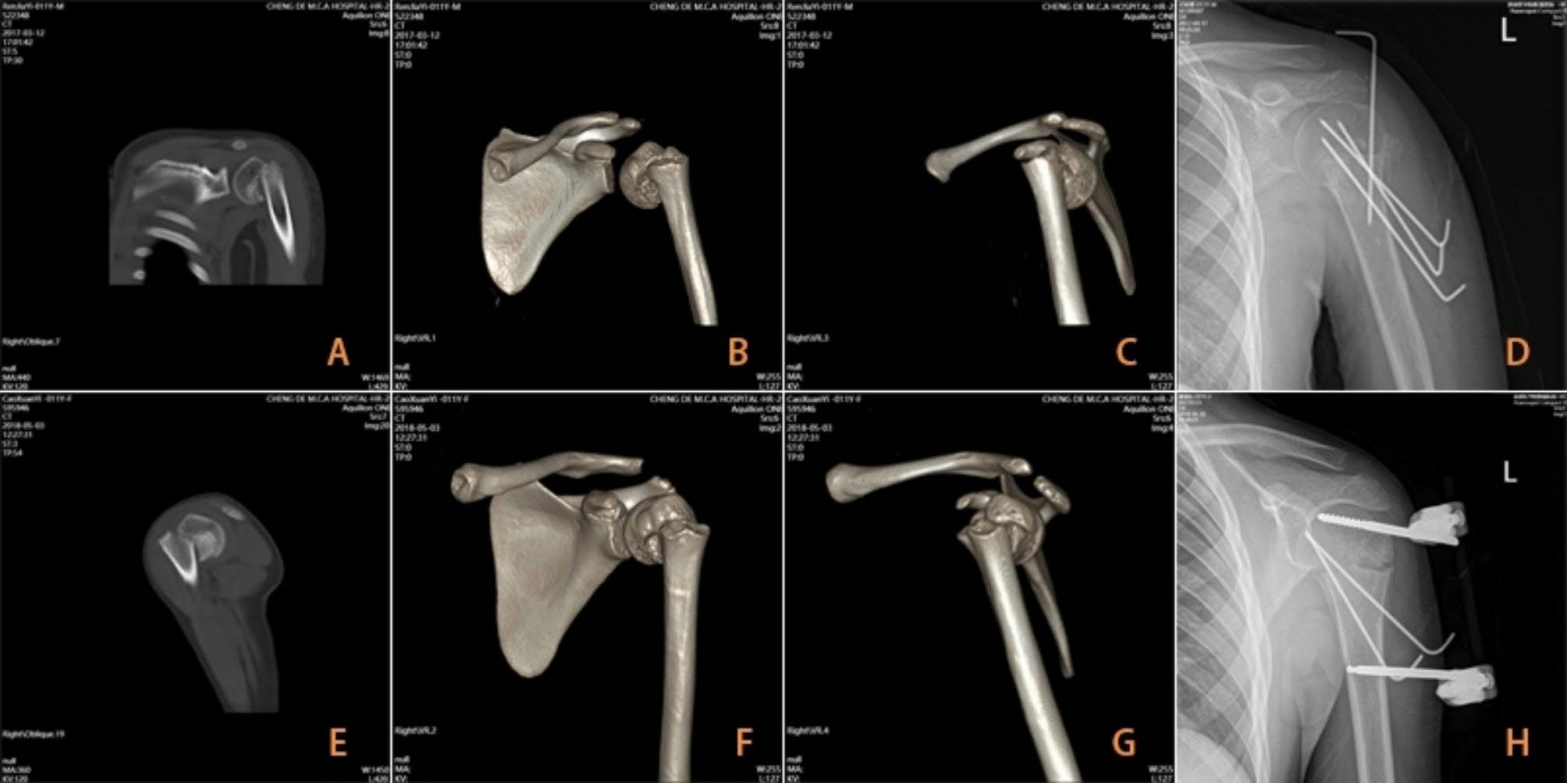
(A) Preoperative 2D-CT of a 11-year-old male patient with N-H IV proximal humeral fracture. (B) and (C) Preoperative 3D-CT of proximal humerus fracture. (D) Postoperative X-ray of proximal humerus fracture with Kirschner wires. (E) Preoperative 2D-CT of a 11-year-old female patient with N-H IV proximal humeral fracture. (F) and (G) Preoperative 3D-CT of proximal humerus fracture. (H) Postoperative X-ray of proximal humerus fracture with the external fixation
